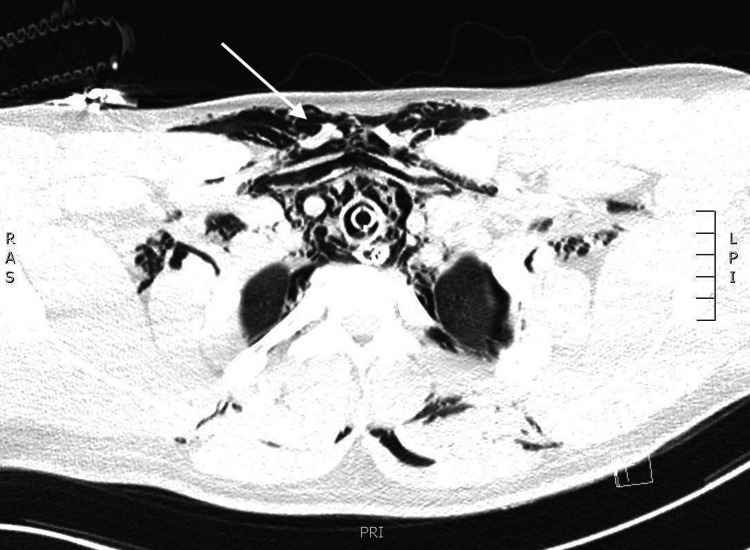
Chest CT highlighting the mediastinal air
